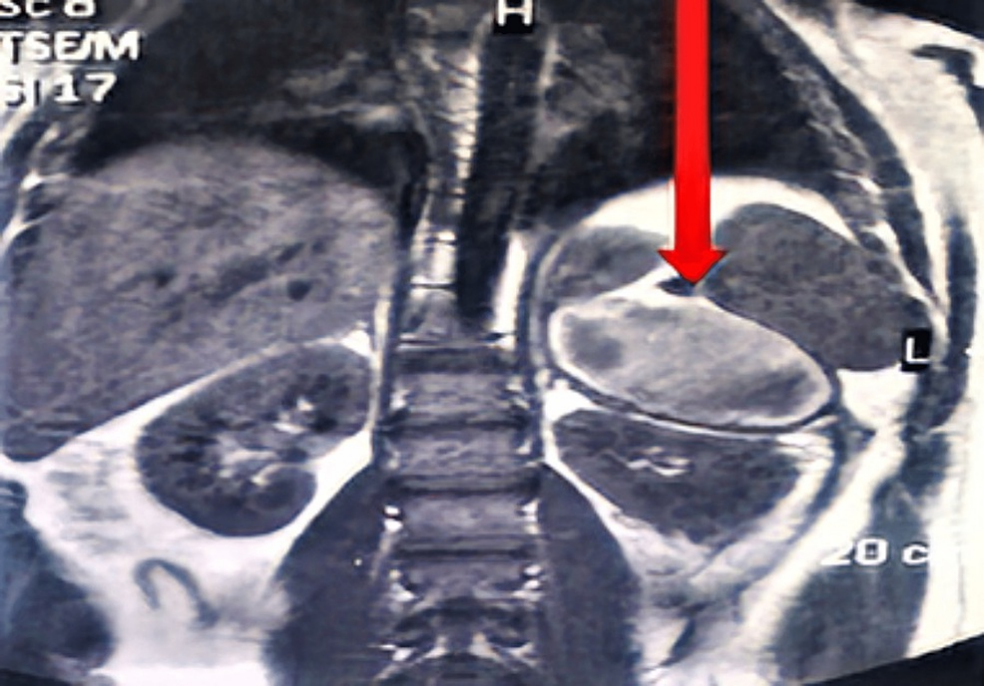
MRI abdomen with adrenal protocol coronal view, showing a heterogenous left adrenal tumour overlying the upper pole of the left kidney (arrow).
